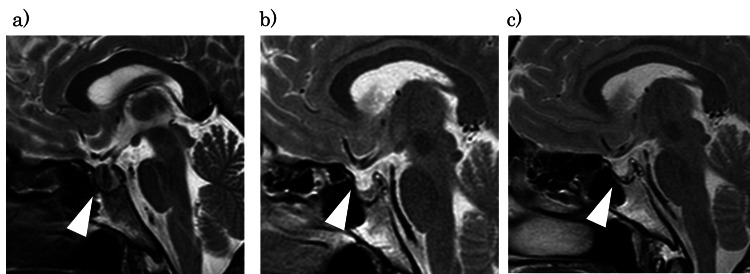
Sequential MRI changes of the pituitary gland in acute Sheehan’s syndrome. (a) Seven days postpartum: marginal enhancement. (b) 2 months: normalization. (c) 6 months: atrophy. The white arrowhead shows the pituitary gland.
